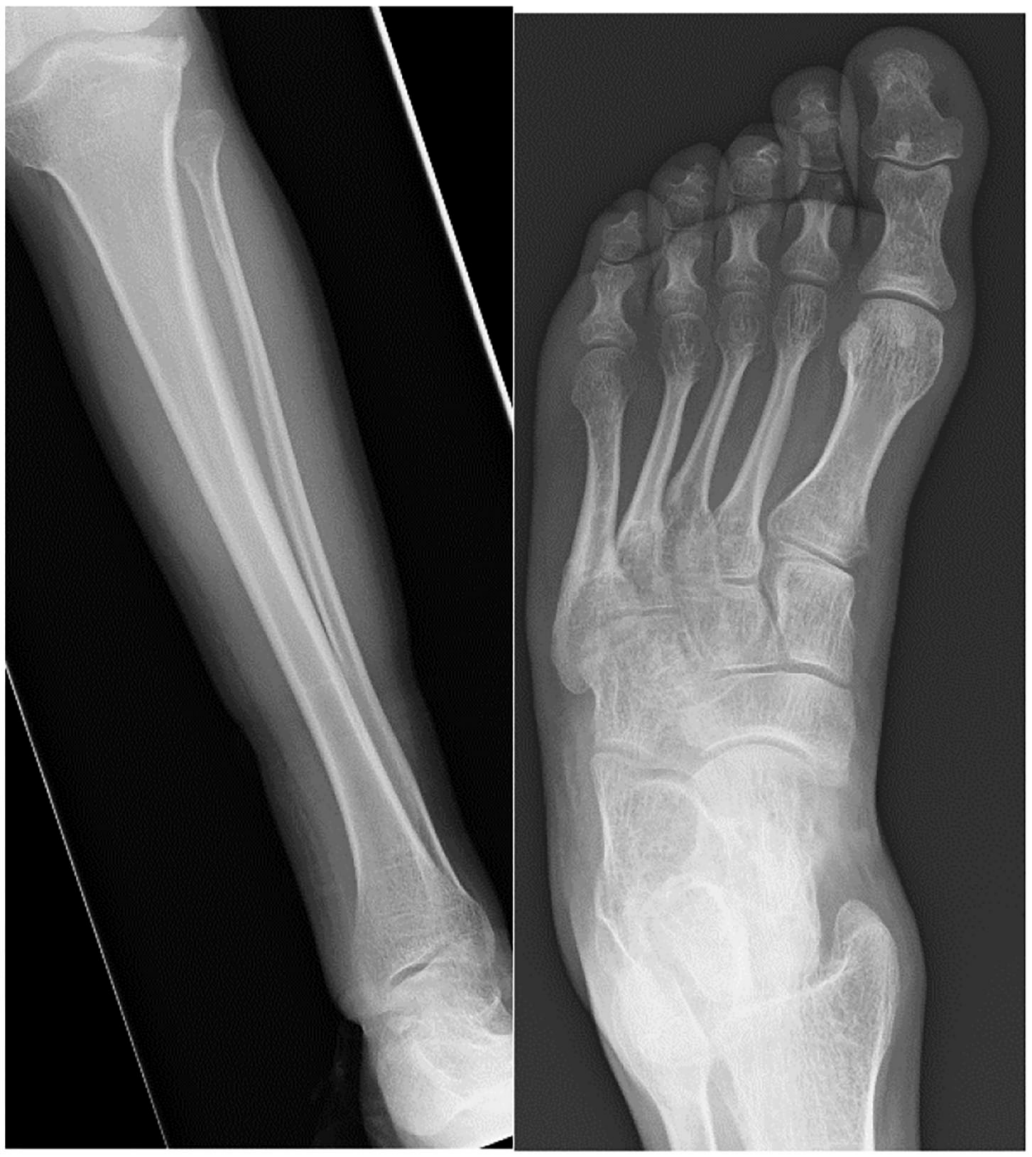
AP radiographs of the tibia and fibula and an AP view of the foot in a 22- year-old male with MPS I. The tibia shows epiphyseal dysplasia on the lateral side of the ankle and fibula hypoplasia causing valgus wedging with a decreased LDTA, tipping the hindfoot into valgus. The foot shows dysplastic and shortened metatarsals with widened proximal metaphyses.
